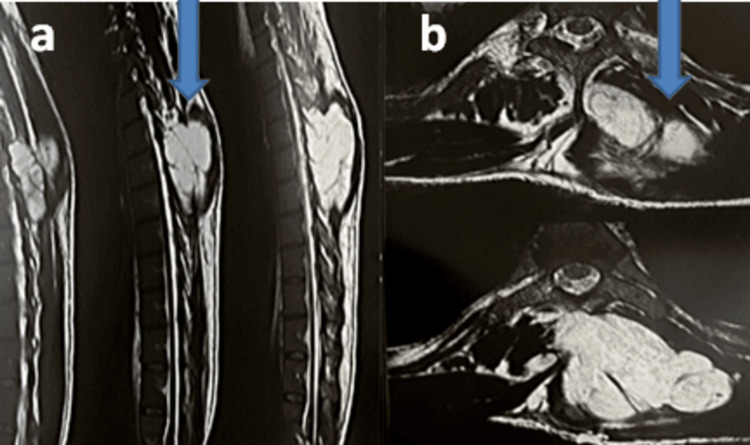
Injected dorsal MRI in sagittal (a) and axial (b) sections Dorsal tumoral process, developing intradurally extramedullarily from D6 to D8, extending to the adjacent left foramina with corresponding costovertebral osteolysis, and significant extension to the paravertebral soft tissues from D4 to D8, with frank T2 hypersignal, first suggesting a schwannoma.
